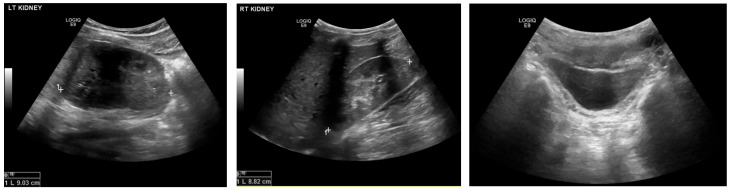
Ultrasound of his kidneys and bladder.
